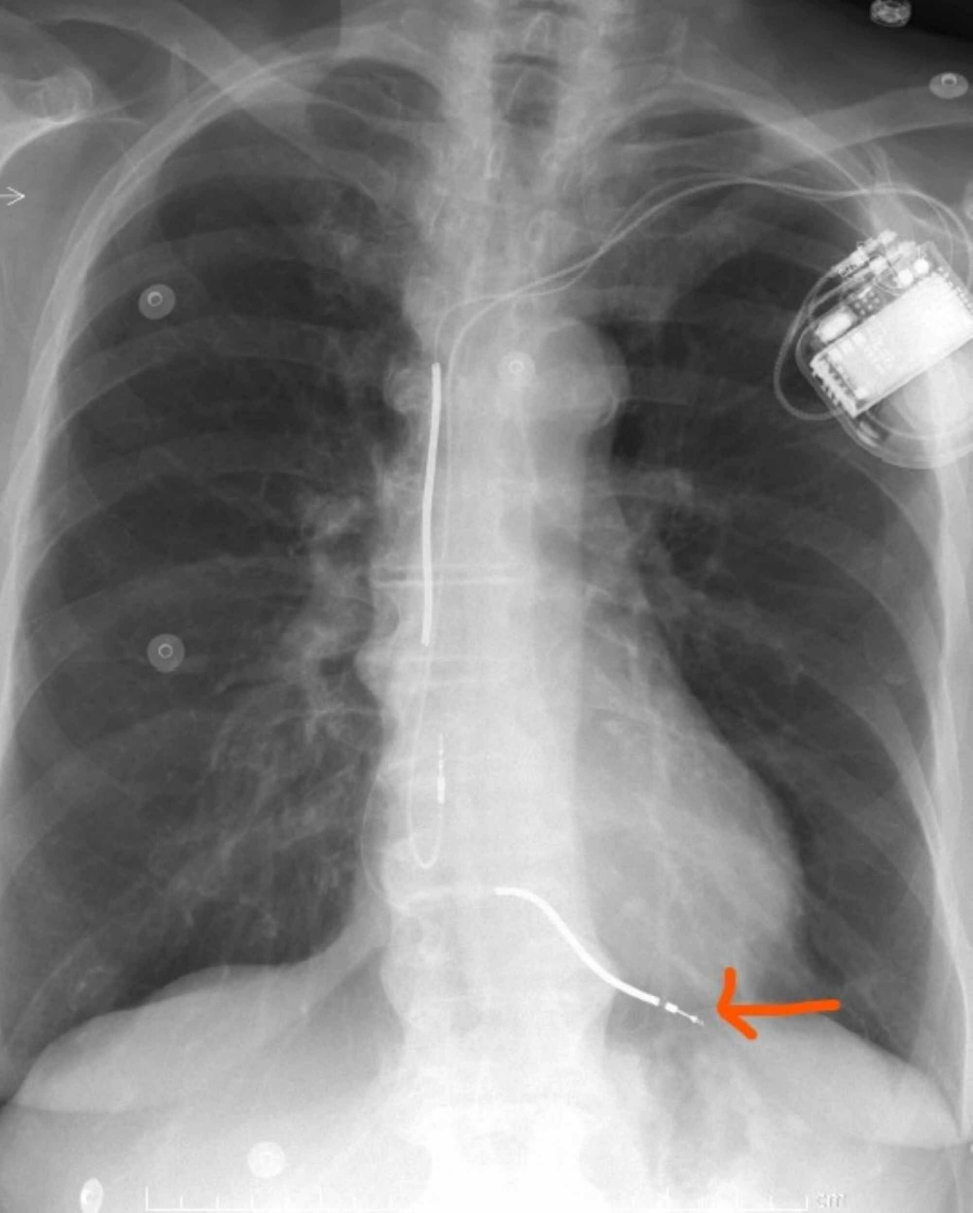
Chest X-ray showing abnormal position of the ventricular lead.
